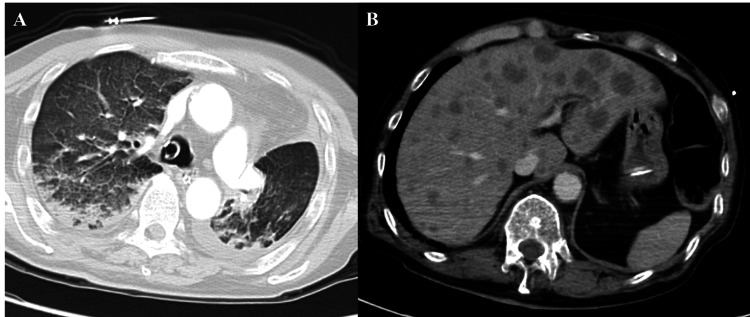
CT findings on admission. (A) An axial chest CT image shows diffuse ground-glass opacities and marked interlobular septal thickening. (B) An axial CT image of the upper abdomen reveals multiple, widespread hypodense lesions throughout the liver, consistent with metastatic disease.
